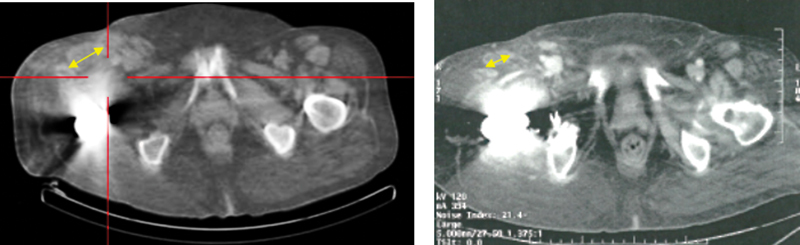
Comparative computed tomography showing the morphological modification in the periprosthetic mass of the right hip and the proximal part of the right thigh (
